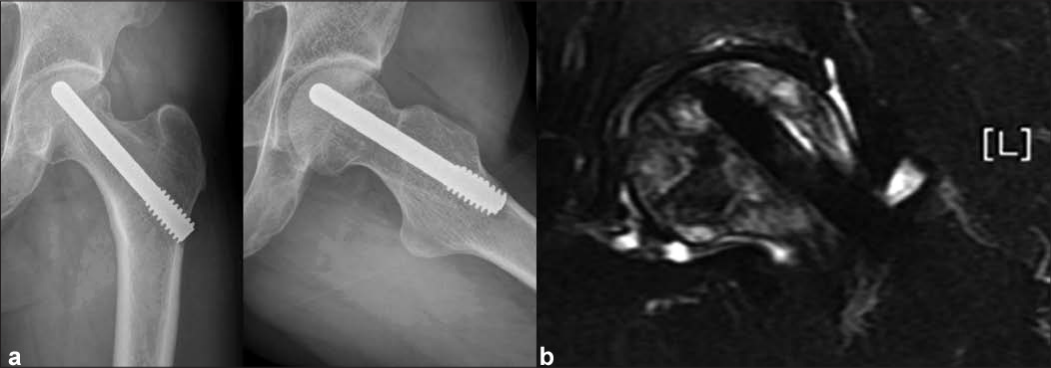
(a) Postoperative radiograph anteroposterior and lateral views showing a well placed implant with no signs of subchondral collapse or depression in the articular surface. (b) Follow-up MRI scan showing porous tantalum rod in the necrotic area, with reactive marrow signal changes around the tip of the implant, without any evidence of femoral head collapse
